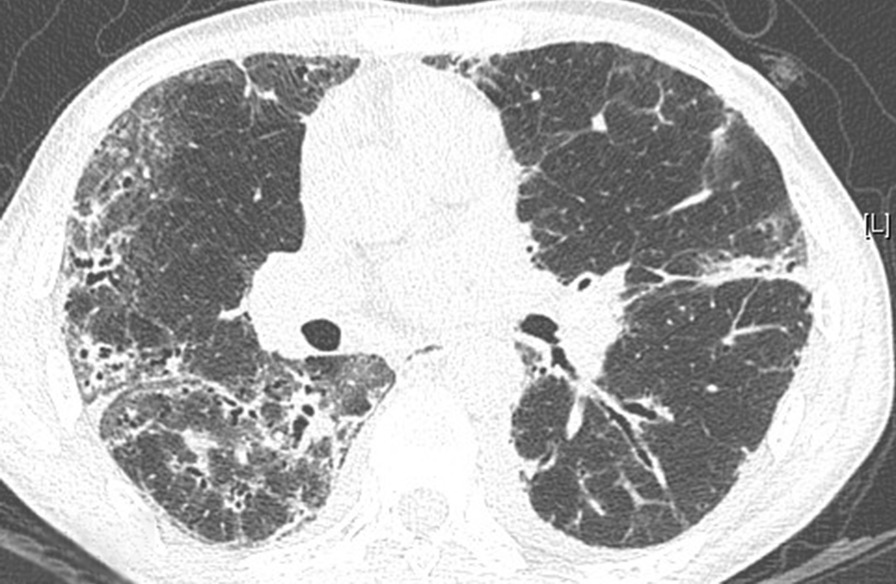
The CT of a male ILD patient in his 50s, who was anti-EJ positive, showing ground glass opacity, and traction bronchiectasis
